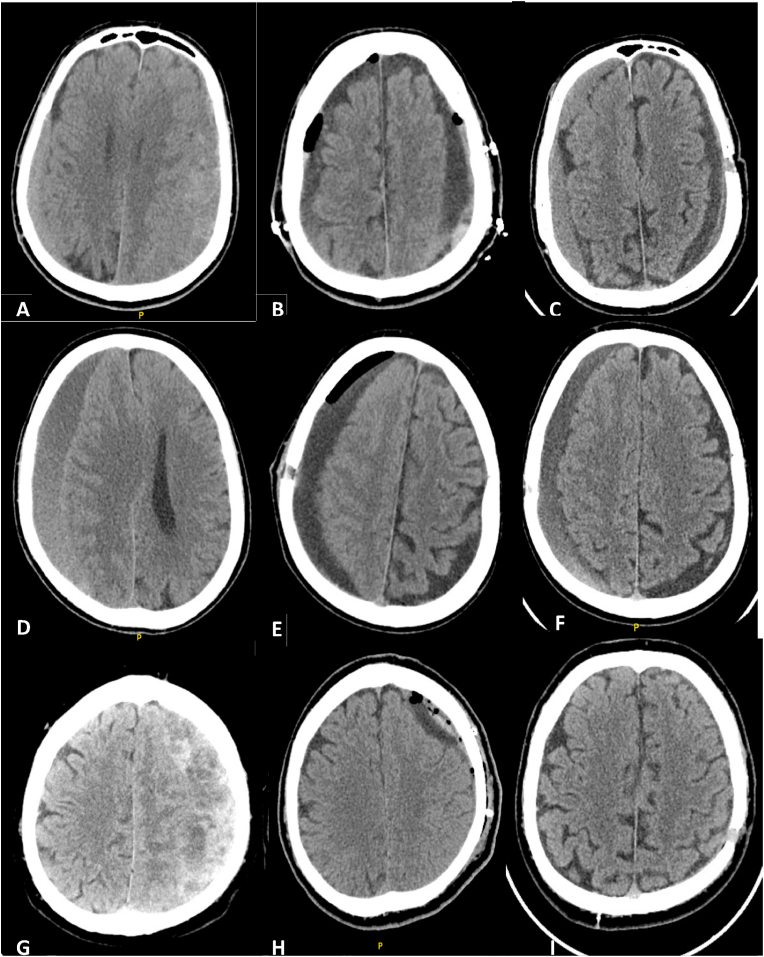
Difference between CT findings preoperatively and postoperatively for three patients. (A) Preoperative CT findings of an 83-year-old male patient with bilateral CSDH. (B) Postoperative CT findings after surgery with a single burr hole on the right and double burr holes on the left, showing good results.(C) CT findings 25 days post-surgery indicating recurrence of CSDH on the left side.(D) Preoperative CT findings of a 75-year-old male patient with unilateral right CSDH and a history of acetylsalicylic acid use.(E) Postoperative CT findings after treatment with a single burr hole surgery.(F) CT findings 36 days post-surgery revealing recurrence of CSDH.(G) Preoperative CT findings of a 60-year-old male patient with unilateral CSDH and membranes. (H) Postoperative CT findings after treatment with a double burr hole surgery. (I) CT findings 60 days post-surgery showing no signs of CSDH recurrence. CSDH- chronic subdural hematoma.
